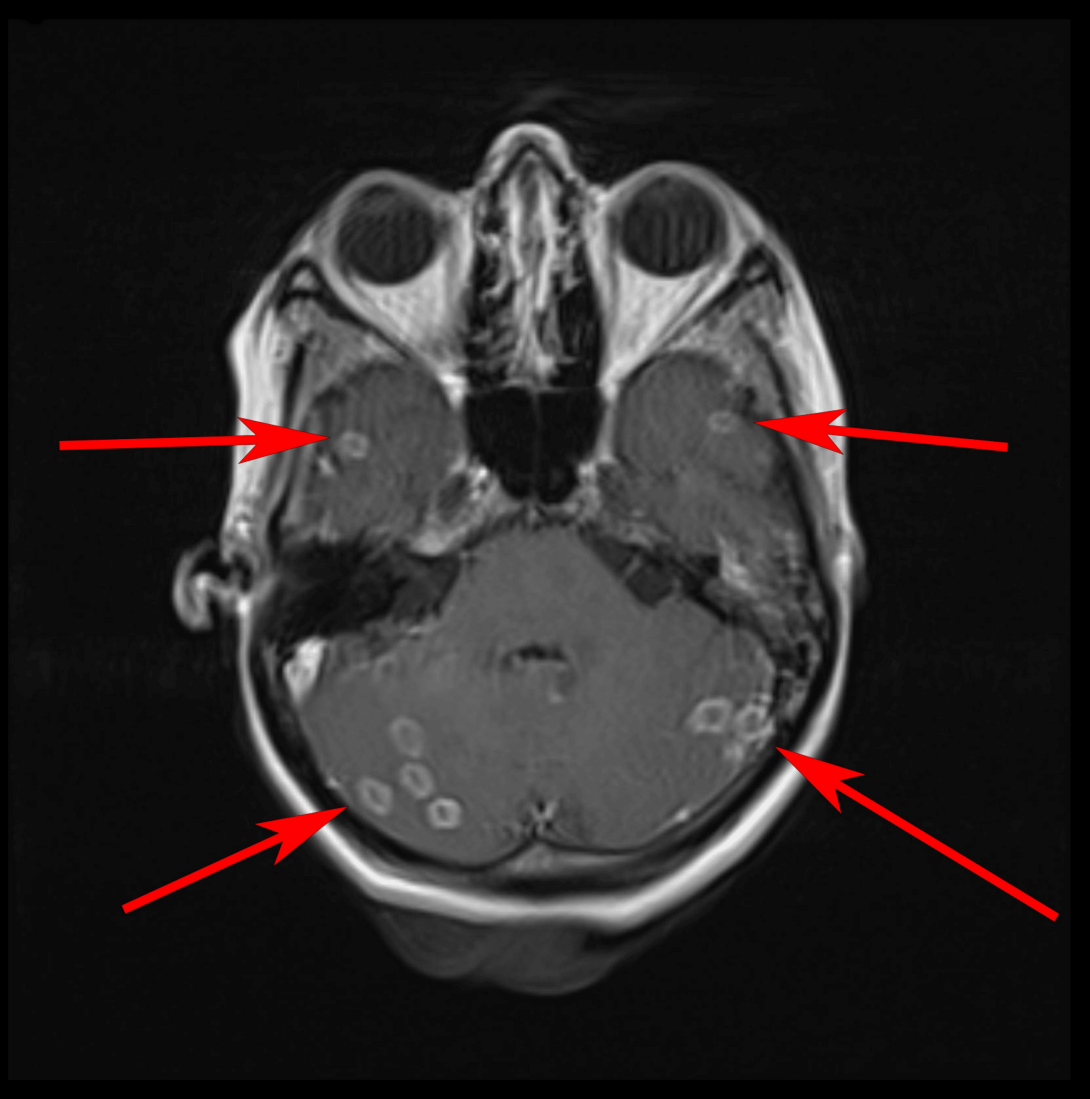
MRI head with arrows pointing to ring-enhancing lesions.
